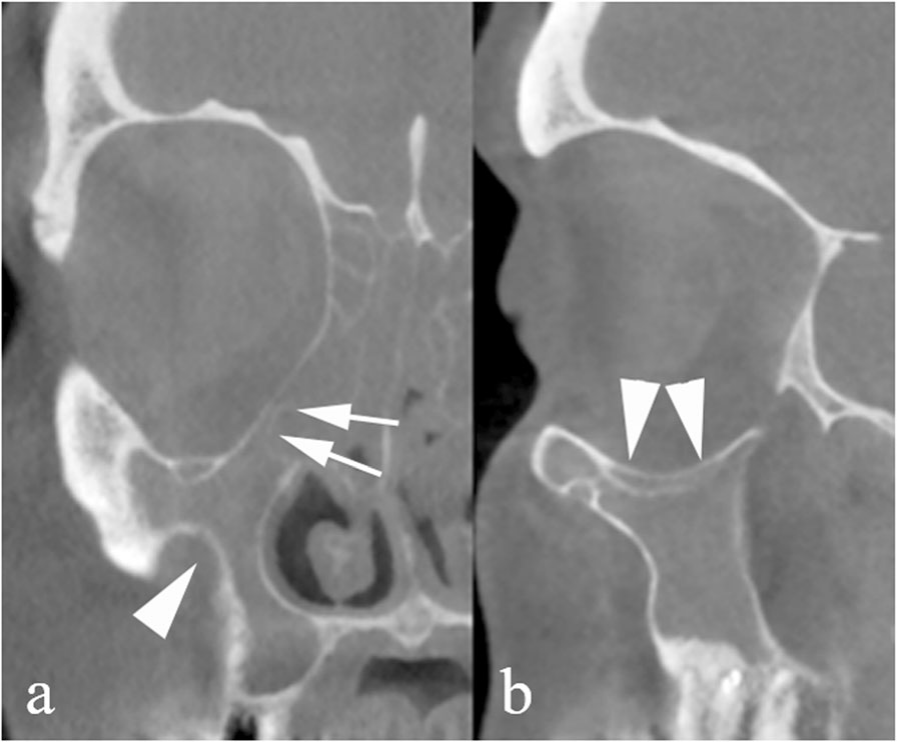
Uncinate process collapse. CBCT reformations on the coronal (a) and sagittal (b) plane. The right uncinate process (arrows) lies in contact with the inferomedial orbital wall. The maxillary sinus and ethmoid infundibulum are opacified by inflamed mucosa and shrinkage of the lumen, and its walls (arrowheads) are remodeled and display an abnormally concave shape. The condition is referred to as silent sinus syndrome
